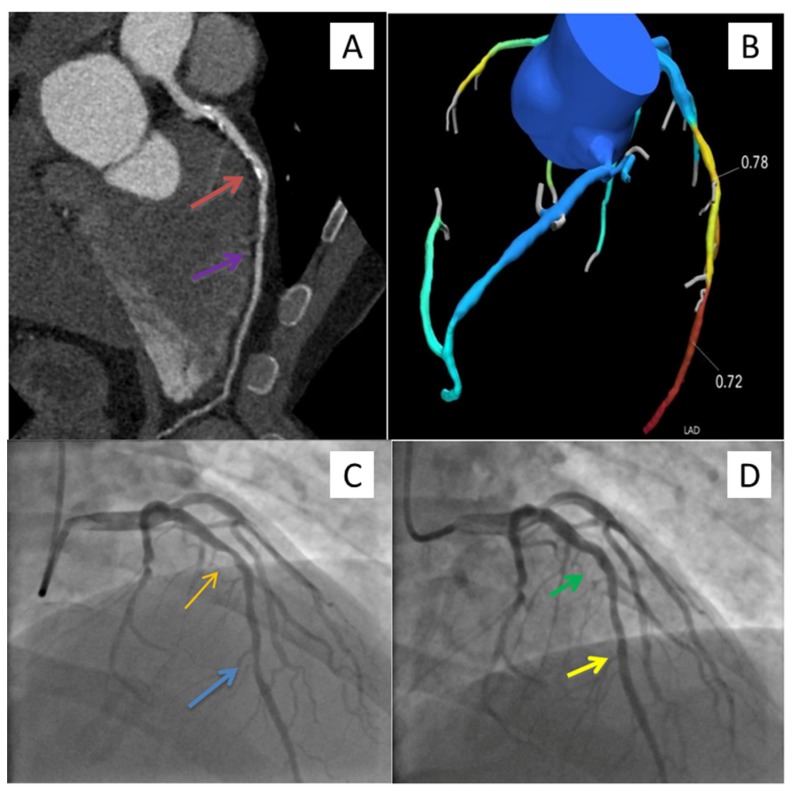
Study patient case. A 48-year-old male with hypertension, diabetes, dyspnea on exertion and atypical chest pain underwent coronary CTA. Multiplanar reformat of coronary CTA of the LAD (A), FFR_CT_ (B), ICA pre- (C) and post- (D) PCI. LAD demonstrated a mid-calcified and non-calcified intermediate (50–70%) stenosis and a distal non-calcified intermediate (50–70%) stenosis (red and purple arrows), with evidence of lesion-specific ischemia. FFR_CT_ values distal to the mid and distal LAD stenoses were 0.78 and 0.72, respectively. The patient underwent successful PCI (green and yellow arrows) of the mid and distal LAD stenoses (orange and blue arrows). FFR_CT,_ fractional flow reserve derived from coronary computed tomography angiography (coronary CTA) datasets; ICA, invasive coronary angiogram; LAD, left anterior descending artery; PCI, percutaneous coronary intervention.
